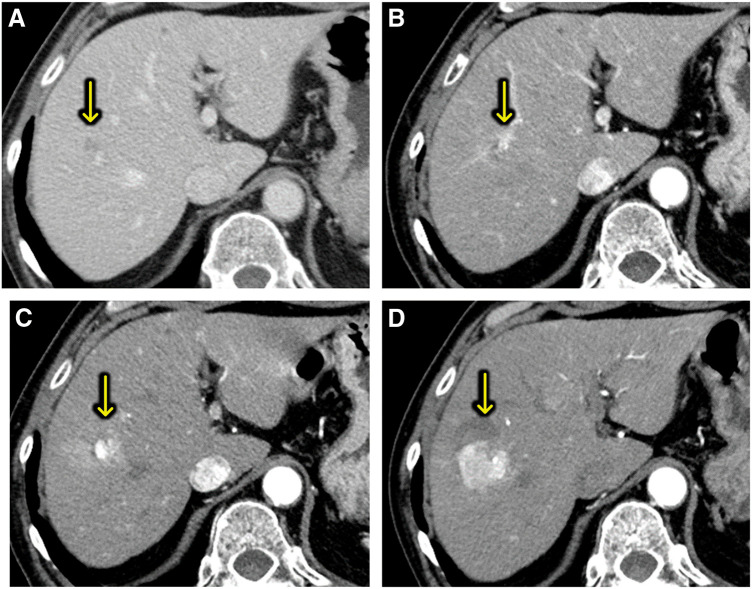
Serial computed tomography (CT) imaging findings of liver tumor. (A) Initial CT imaging revealed a low-attenuation nodule in segment 8. (B) After 2.5 years, the lesion showed early enhancement and measured 14.2 mm, with a doubling time of 436 days. (C) At 4 years, the tumor further increased in size to 17.6 mm and demonstrated early arterial enhancement and washout. (D) By the sixth year, the tumor had grown to 40.6 mm, with heterogeneous enhancement and evidence of vascular penetration through the tumor.
